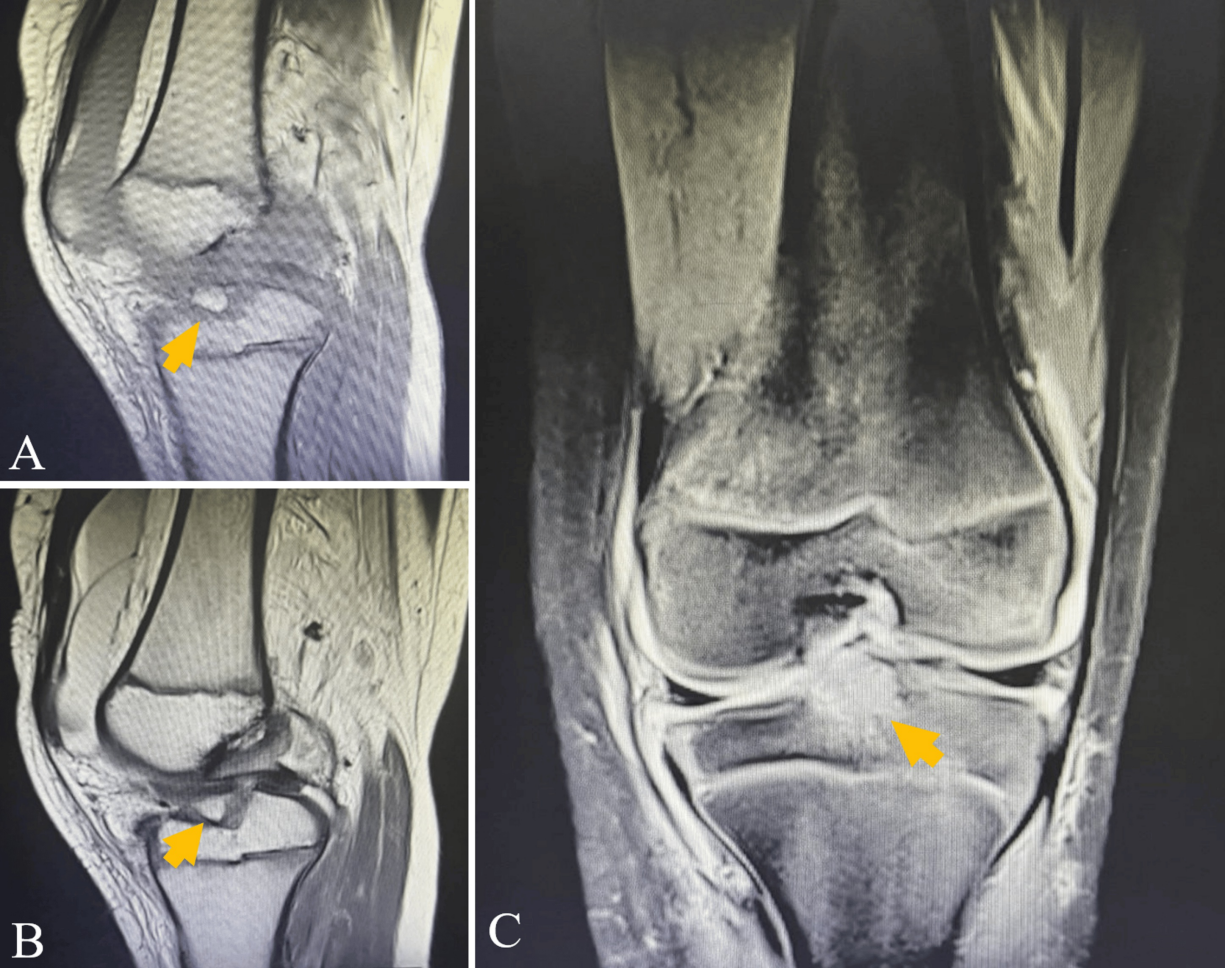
MRI findings MRI (T2 fat-suppressed/STIR) demonstrates bone contusions (arrow) as a hyperintense marrow signal and an avulsion of the ACL tibial insertion with elevation of the anterior tibial eminence, without disruption of the ACL fibers, on sagittal (A, B) and coronal (C) images MRI: magnetic resonance imaging; STIR: short TI inversion recovery; ACL: anterior cruciate ligament
